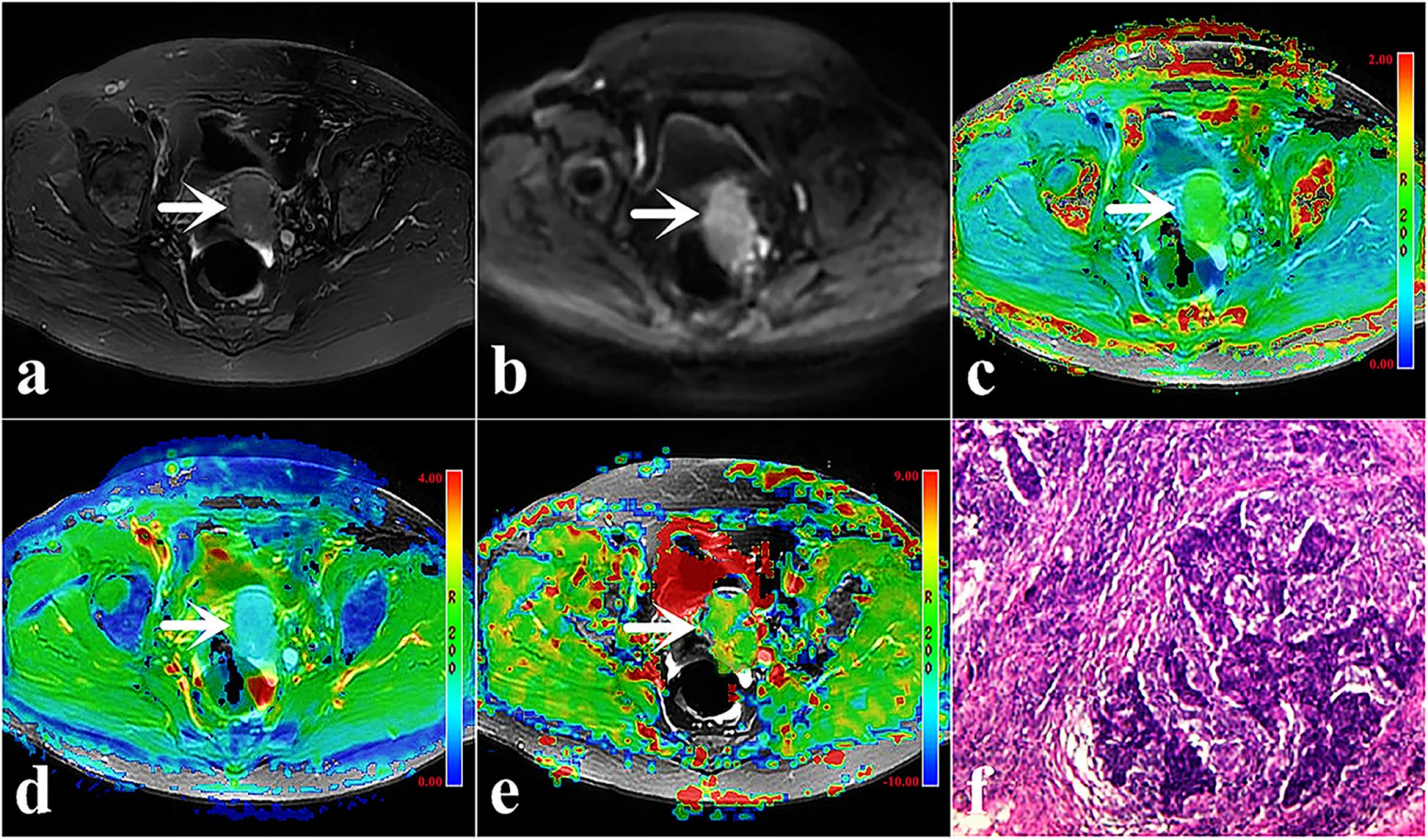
Grade 3 of CSC in a 42-year-old woman (arrowheads), ADC = 0.94 × 10^− 3^/mm^2^, MK = 0.90, MD = 1.03 × 10^− 3^/mm^2^, and MTRasym = 3.07%. a Map of T2WI, b Map of DWI (b = 1000 s/mm^2^), c Pseudo colored maps of MK, d Pseudo colored maps of MD, e Pseudo colored maps of MTRasym, f Pathological images (original magnification, × 100)
